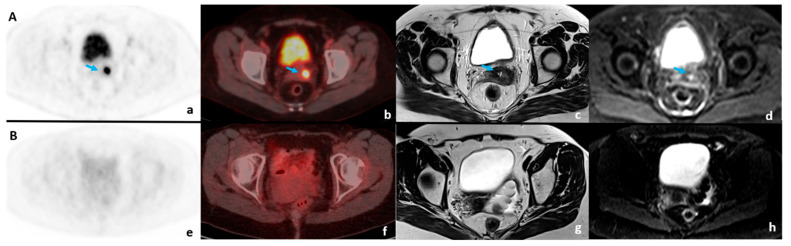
Concordant results of early CCRT posttreatment 18F-FDG PET/CT and MRI examinations are presented in row (A) (PR) and row (B) (CR). In row (A), residual cancer presence within a partial response is marked with a blue arrow on PET (a), fused PET/CT (b), axial T2w (c), and DWI (b = 800 s/mm^2^) (d) images. In row (B), complete therapy response is noticeable on PET (e), fused PET/CT (f), T2w (g), and DWI (b = 800 s/mm^2^) (h) images in the axial plane.
